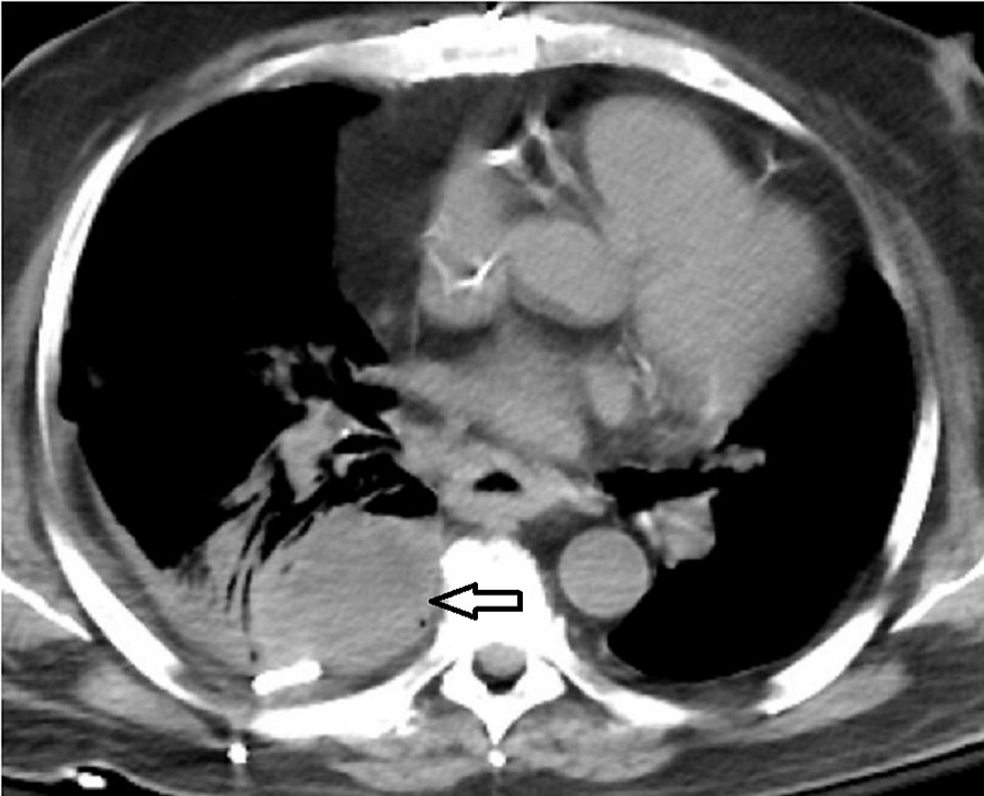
Follow-up CT showing persistent loculations in the right pleural space. CT: computerized tomography
